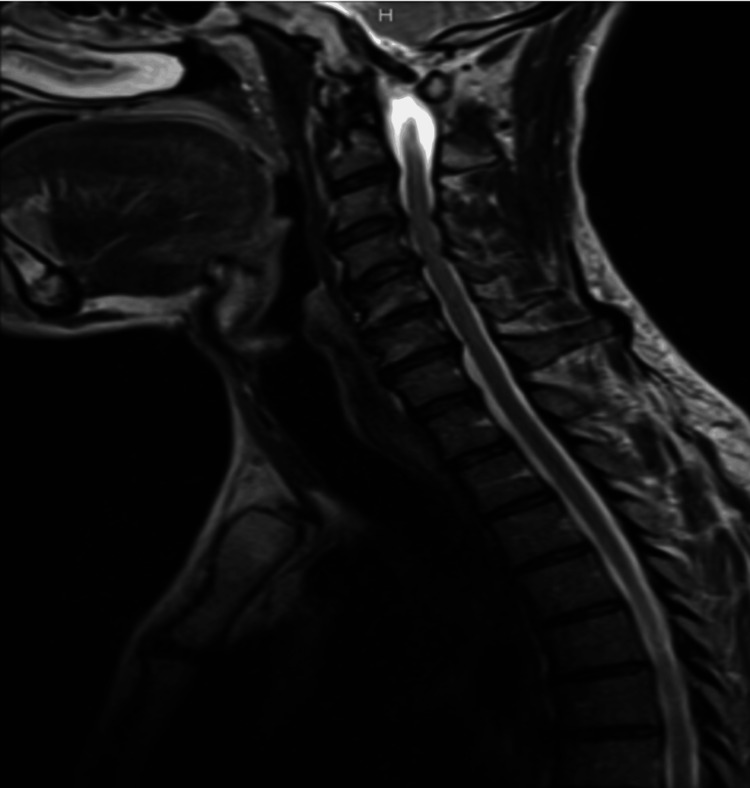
MRI of cervical spine indicating moderate bilateral foraminal stenosis at C5-C6 and C7-T1, with moderate right foraminal stenosis at C3-C4. Mild-to-moderate bilateral foraminal stenosis was observed at C6-C7. MRI: Magnetic resonance imaging; C3: 3rd cervical vertebra; C4: 4th cervical vertebra; C5: 5th cervical vertebra; C6: 6th cervical vertebra; C7: 7th cervical vertebra; T1: 1st thoracic vertebra
